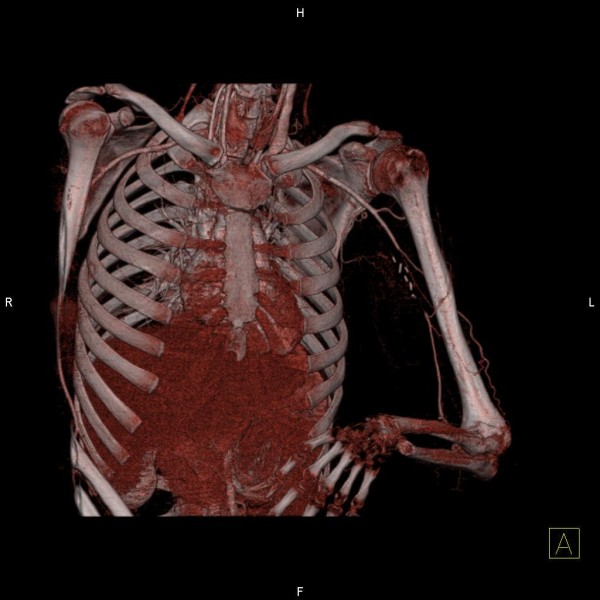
Ten days postoperative three-dimensionally reconstructed angio CT scan. Postoperative view of the three-dimensionally reconstructed angio CT scan 10 days after the removal of the pseudoaneurysm. Intact distal flows were noted.
